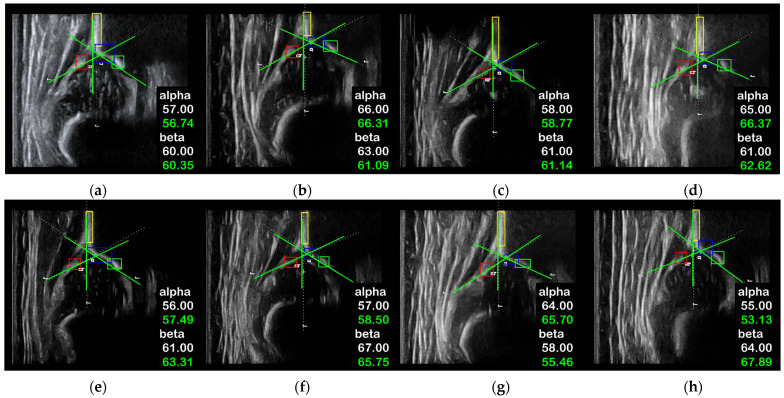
Qualitative comparisons for the angle measurement between the SM mode and the sonographers. The white dash lines are the manual plot in clinic. The green lines are formed by the SM mode based on the detected bounding boxes. The white values are given by sonographers, and the green values are given by the SM mode. The absolute differences between SM mode and sonographers are (a) Δα = 0.26°, Δβ = 0.35°; (b) Δα = 0.31°, Δβ = 1.91°; (c) Δα = 0.77°, Δβ = 0.14°; (d) Δα = 1.37°, Δβ = 1.62°; (e) Δα = 1.49°, Δβ = 3.31°; (f) Δα = 1.50°, Δβ = 1.25°; (g) Δα = 1.70°, Δβ = 2.54°; (h) Δα = 1.87°, Δβ = 3.89°.
